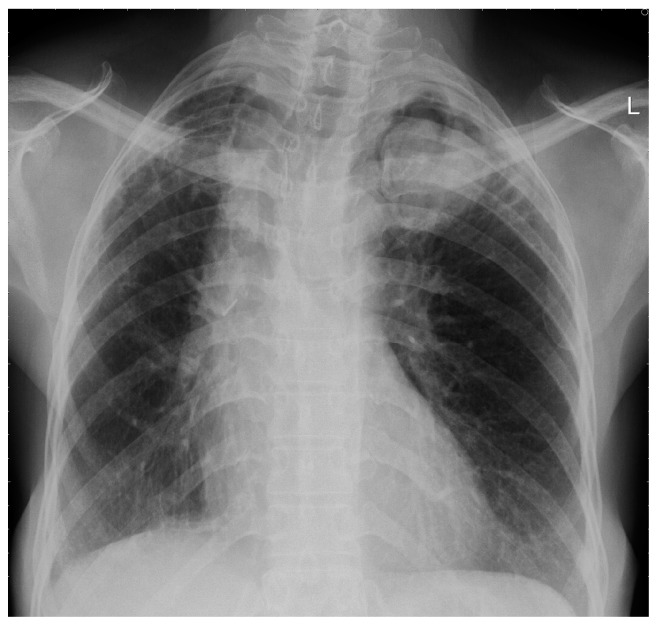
Solitary aspergilloma—Chest X-ray (CXR) demonstrating a left upper lobe aspergilloma in a patient with sarcoidosis.
